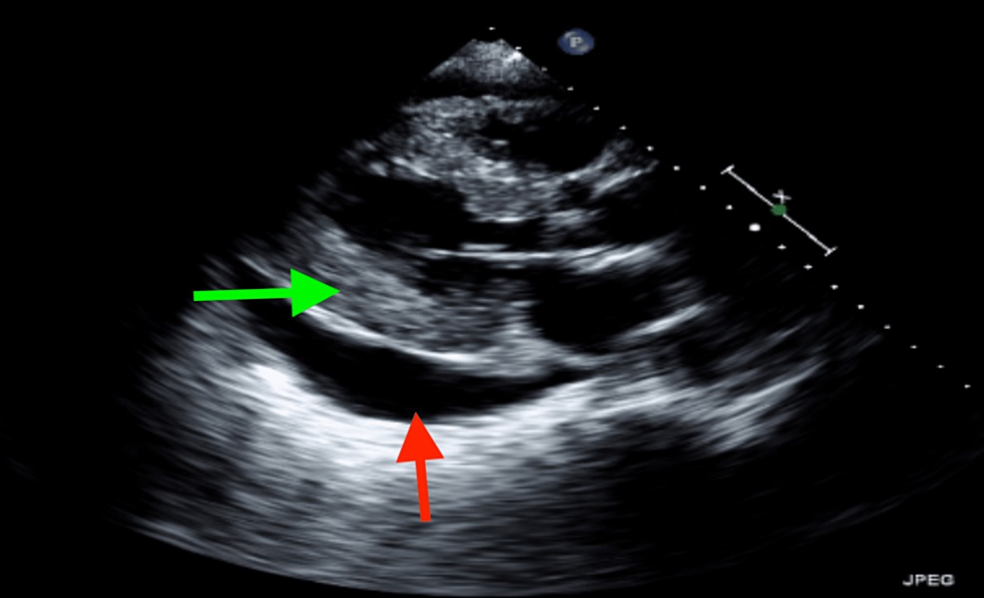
Transthoracic echocardiogram, parasternal long axis view, at the time of admission demonstrating large pericardial effusion at the LV posterior wall and RV free wall and LV hypertrophy The red arrow points to the pericardial effusion. The green arrow points to the left hypertrophied ventricle.
